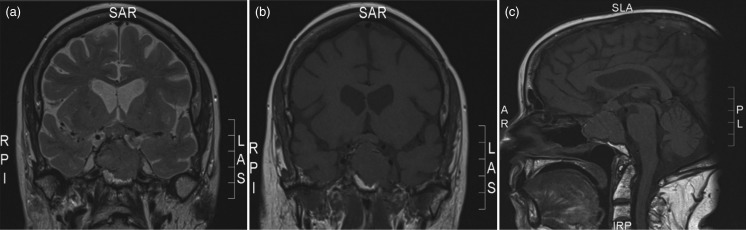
2011 MRI pituitary: (a) coronal T2WI, (b) coronal T1WI and (c) sagittal T1WI respectively.
